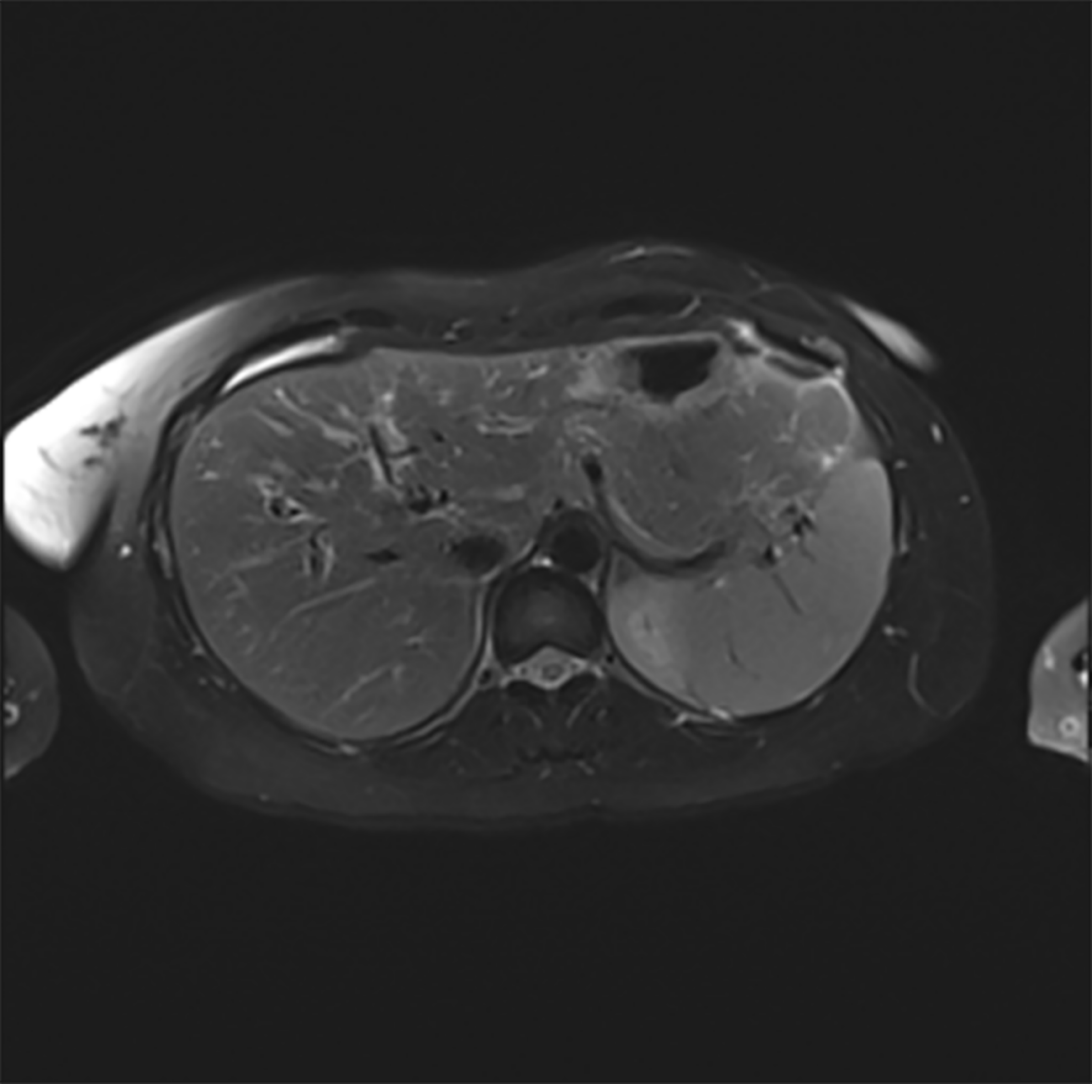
T2-weighted MRT scan of the liver (transverse section) showing normal liver parenchyma and normal biliary ducts.
